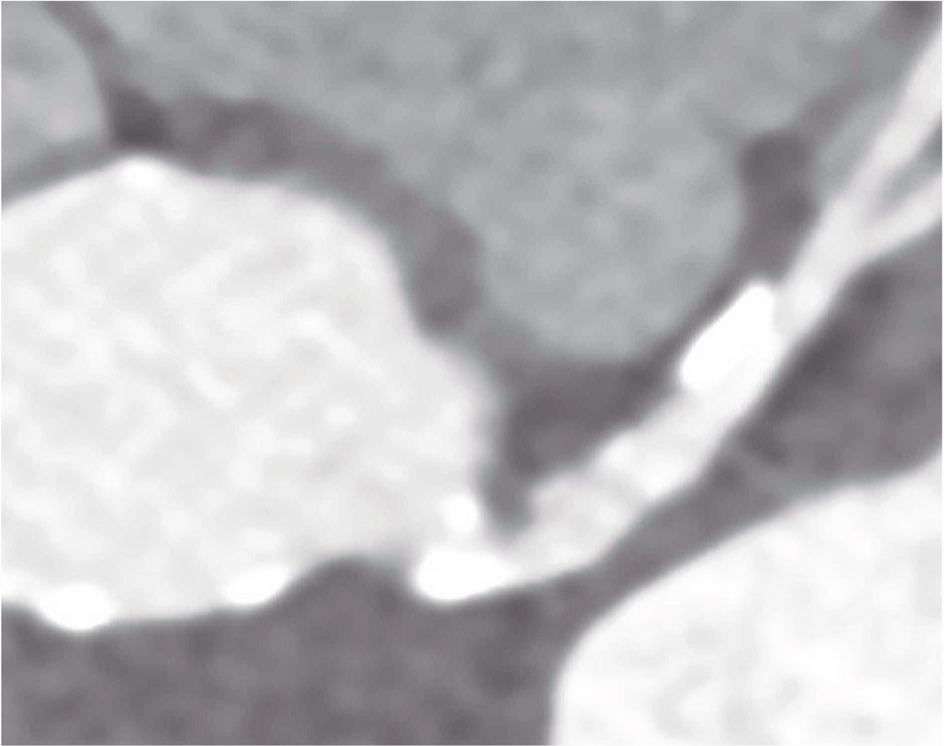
Computed tomography showing coronary atherosclerosis in the left main and the left anterior descending artery.
